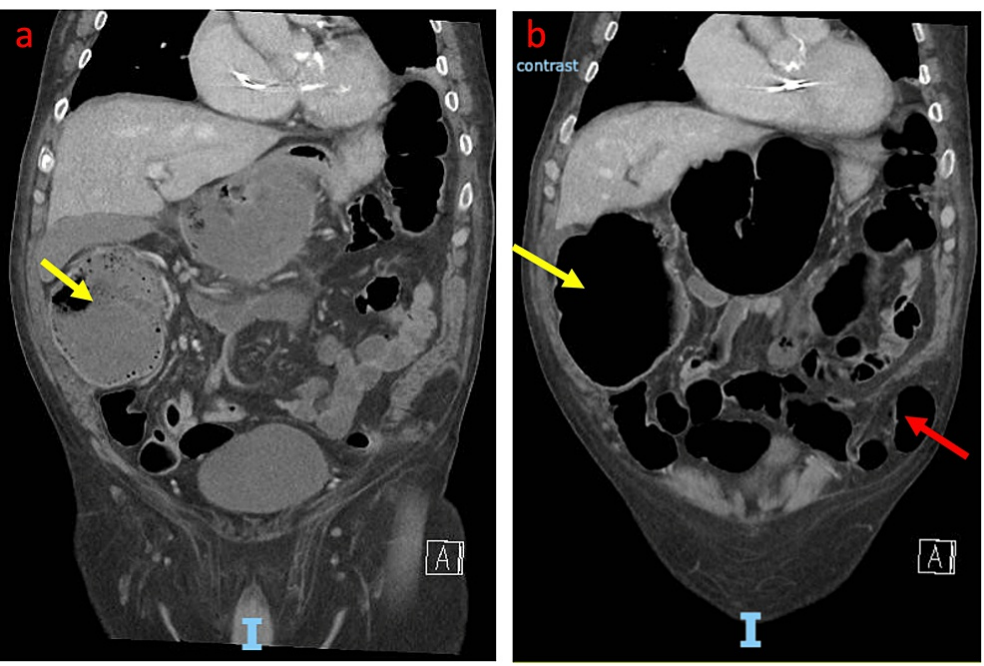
a and b: Coronal CT abdomen and pelvis with IV contrast confirming a large bowel obstruction with cecal dilatation, pneumatosis (yellow arrow), and Spigelian hernia containing the sigmoid (red arrow)
